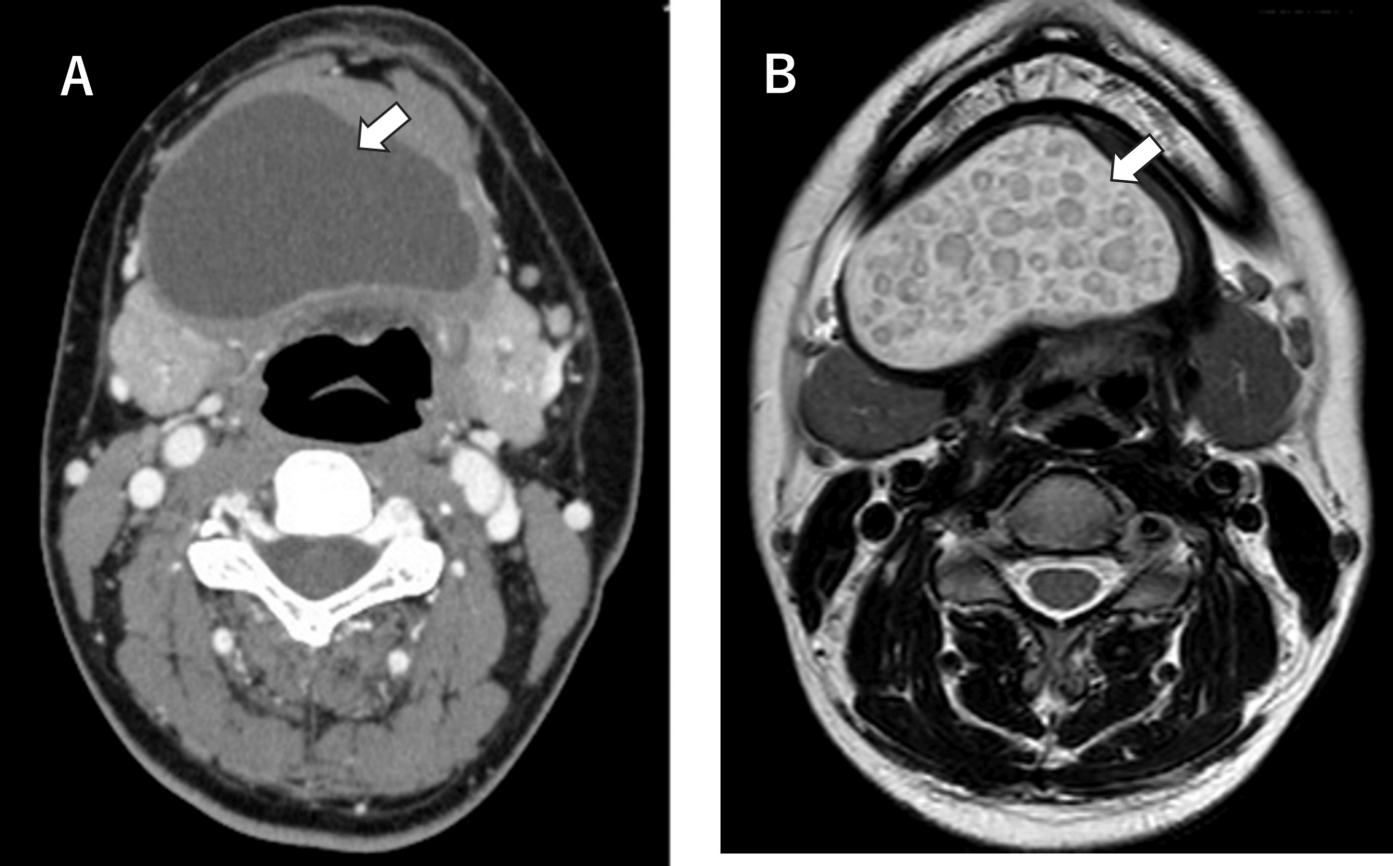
Preoperative computed tomography (CT) (A) and T2-weighted MRI axial view (B) of Case 1 demonstrating the extent of the cyst (arrows). The MRI shows the characteristic "sac of marbles" sign, a radiologic hallmark suggestive of an epidermoid cyst.
